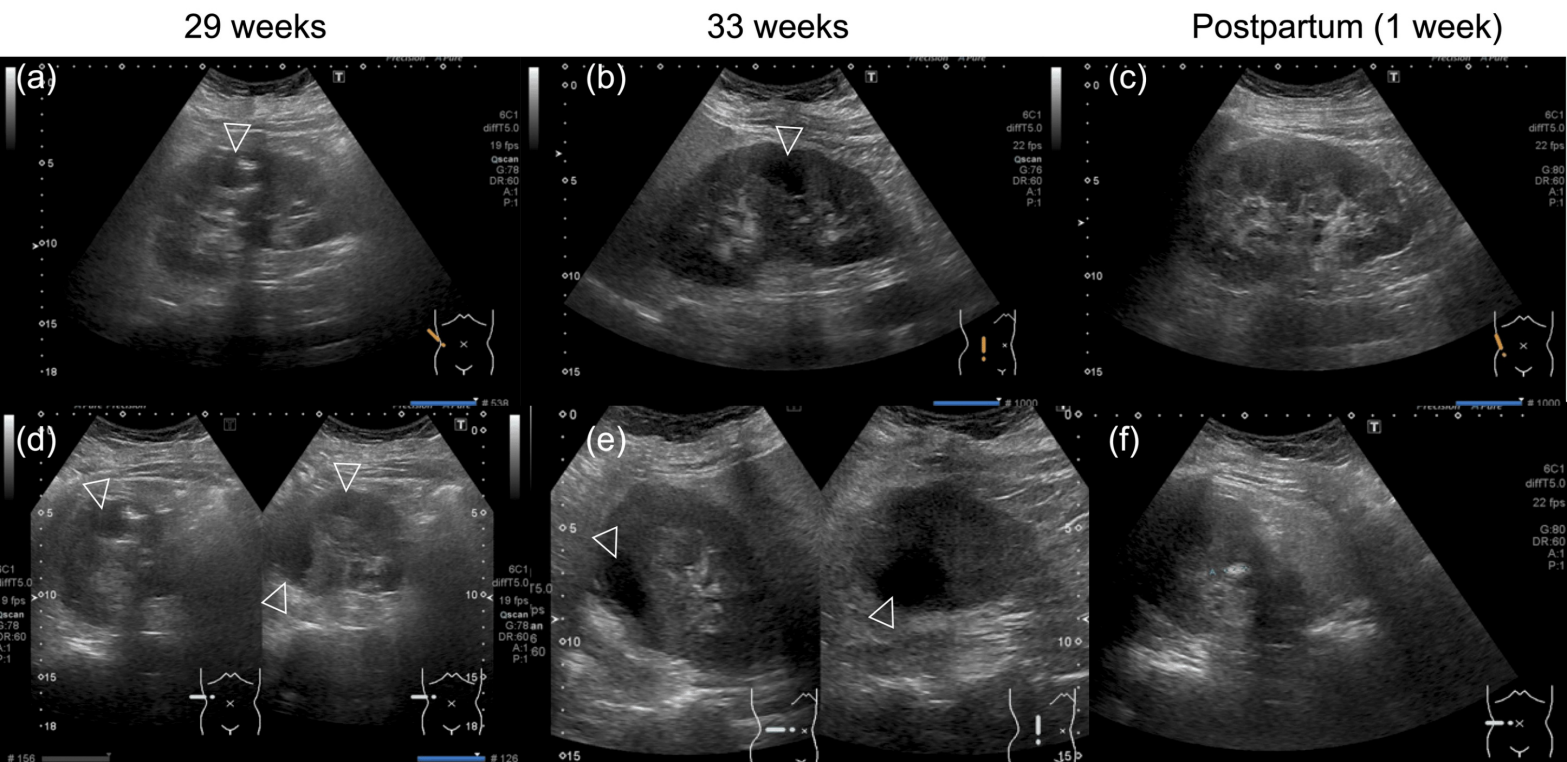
Ultrasound images of emphysematous pyelonephritis Heterogeneous mass mixed with hyperintense and hypointense areas on long-axis (a-c) and short-axis (d-f) ultrasound images. Ultrasonography confirmed renal abscesses (arrowheads) at 29 weeks of gestation (a,d). The abscess was also detected at 33 weeks of gestation (b,e) but gradually shrank and resolved after birth (c,f).
